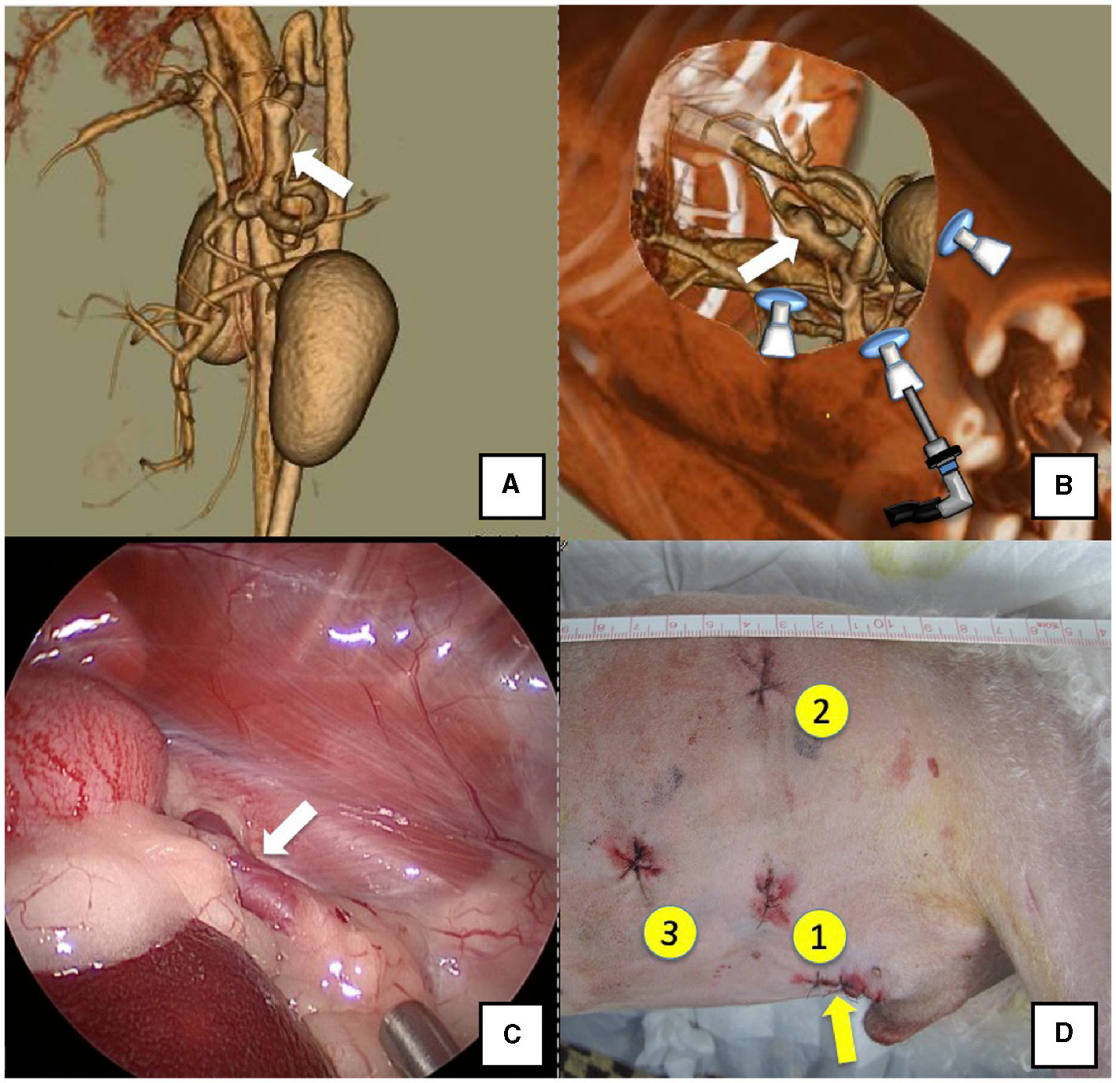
Spleno-azygos shunt (white arrow). (A) 3D-CT angiography image. (B) 3D-CT image of the port locations from reconstructed right recumbent CT images. (C) Laparoscopic image of the shunt vessel and approach. (D) Actual surgical wounds and port placement order. Yellow arrow: location where the LAP PROTECTOR was placed. 3D-CT, three-dimensional-computed tomography.
